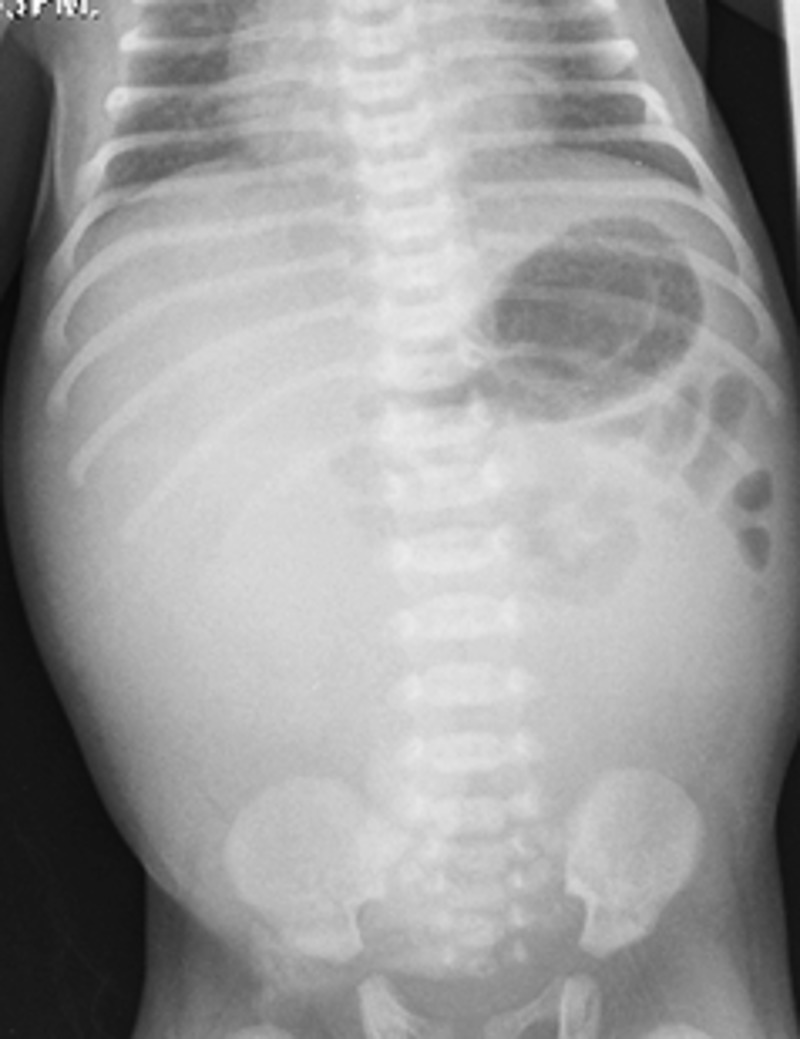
Figure 1: Plain X-ray of abdomen performed at 8 hrs. of age showing mass effect on the right side pushing bowel loops towards the left side with no free air in the peritoneal cavity.
